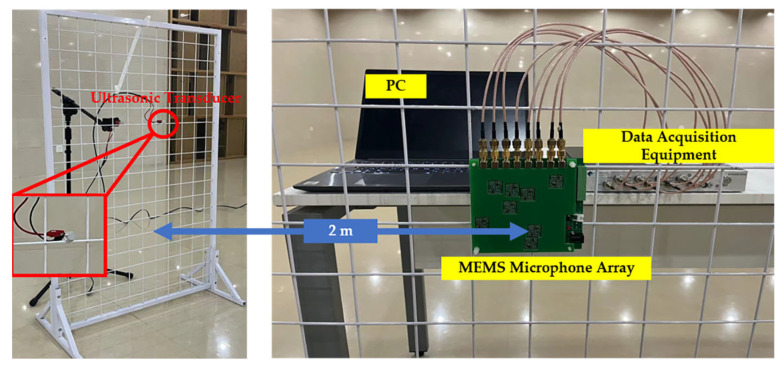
The setup of acoustic source localization experiment. The acoustic wave is produced by an ultrasonic transducer shown in the left picture. The data acquisition system is shown on the right side of the figure.
